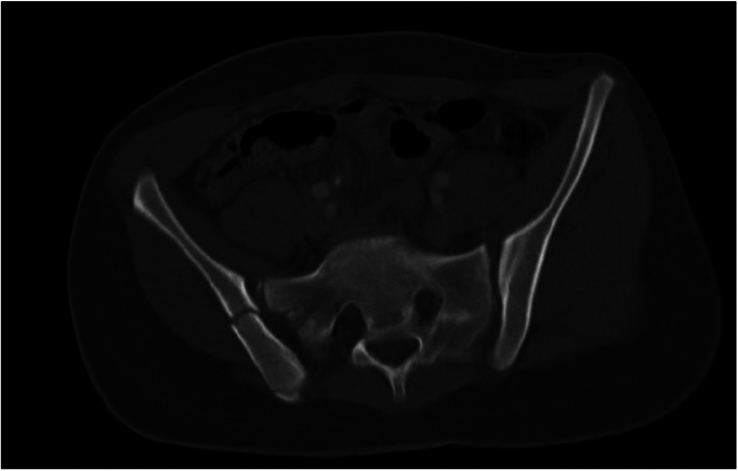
This CT scan shows a pediatric LC3 (lateral compression type 3) fracture pattern in the right hemipelvis. The image highlights a sacral fracture in zone 2, accompanied by a vertical iliac fracture and widening of the sacroiliac (SI) joint. This combination of injuries is characteristic of a pediatric LC3 fracture, indicating significant lateral compression trauma in a pediatric patient.
